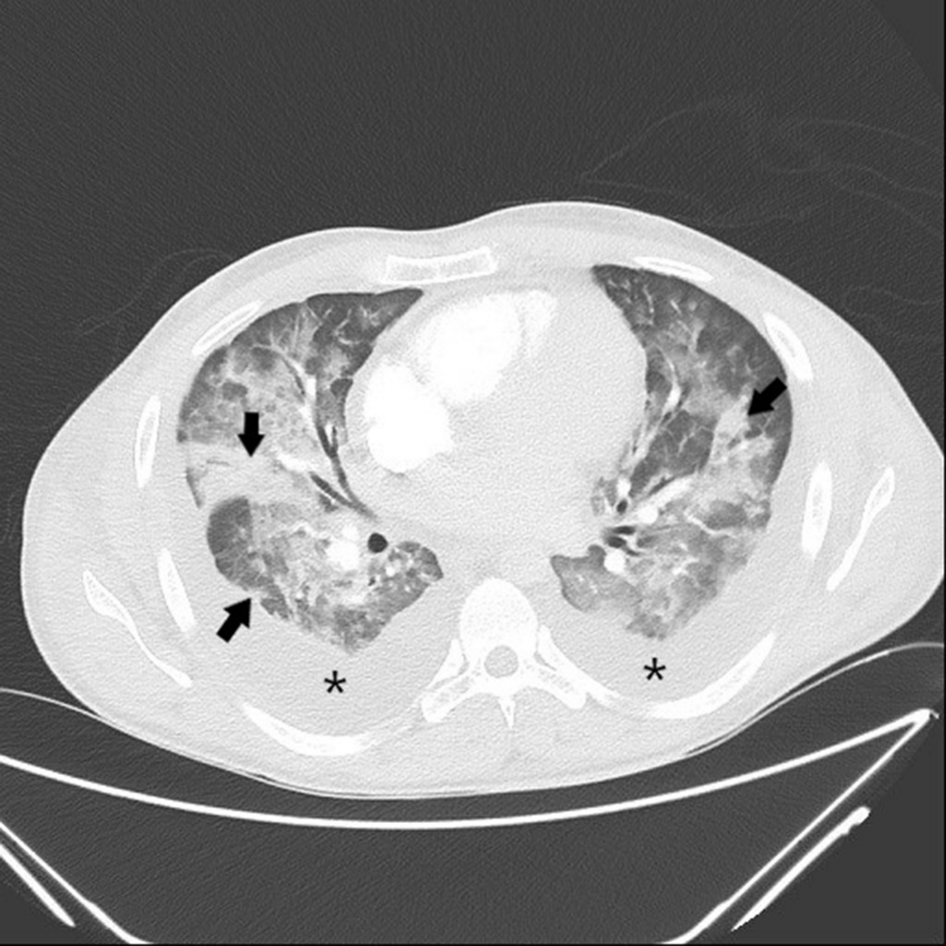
Axial contrast-enhanced CT scan of the chest shows bilateral pleural effusion (*) and multifocal patchy consolidations (black arrows).
